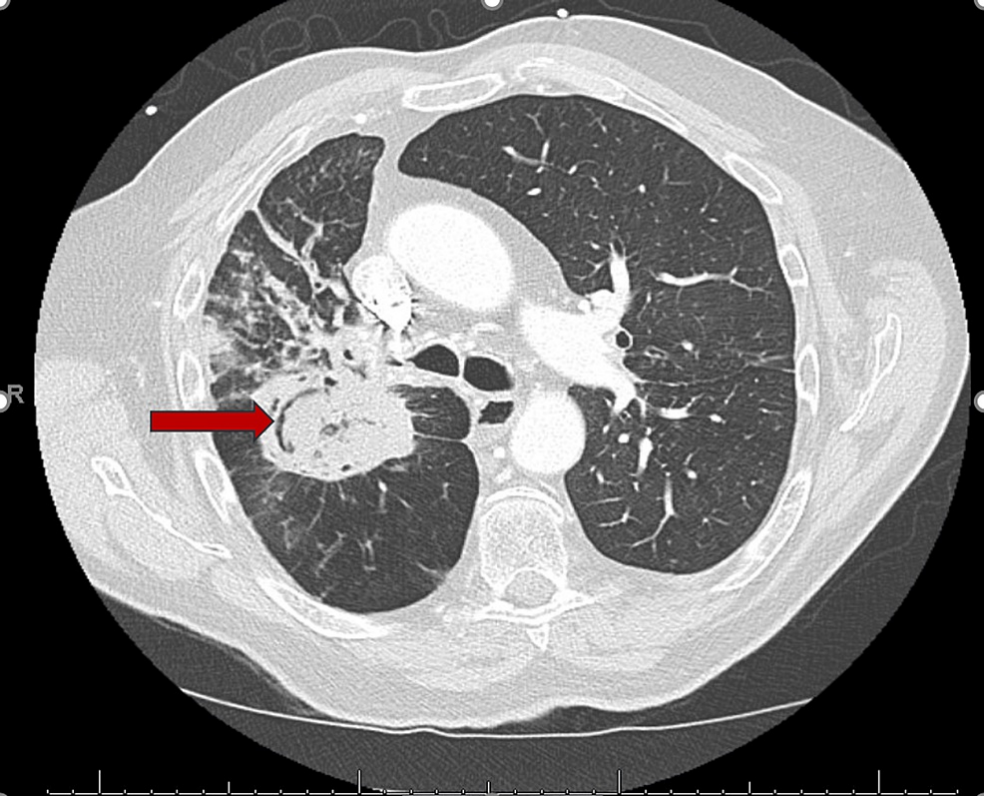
Chest CT scan demonstrating 6 cm aspergilloma (red arrow)
